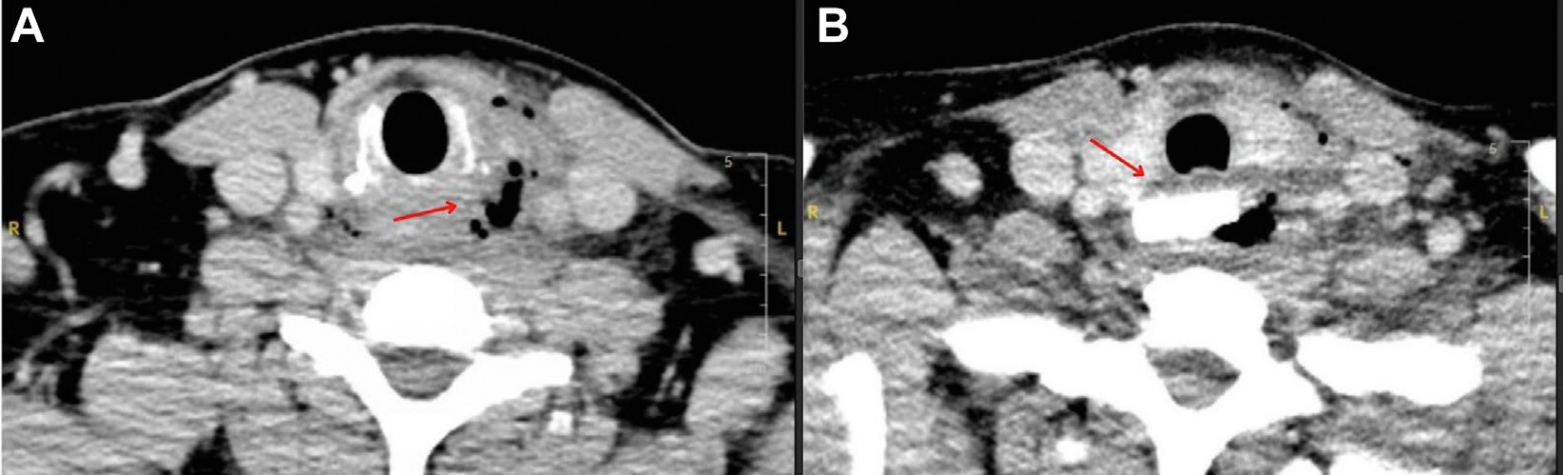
Computed tomography of the neck shows cross-sections demonstrating (A) anterior subcutaneous gas correlating with ultrasound image (Fig 1) and (B) rectangular metallic density.
